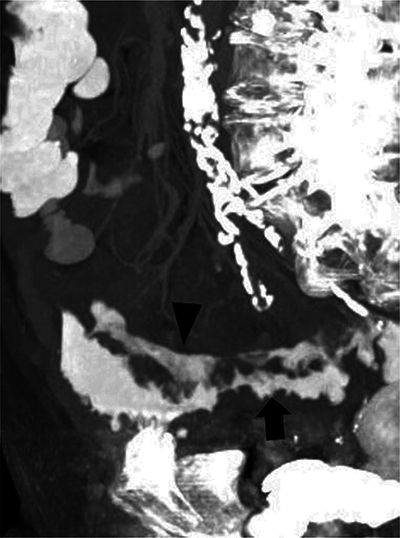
Maximum intensity projection imaging of the abdominal CT: two contrast trajectories are distinguishable, the intramural longitudinal fistula (arrowhead) and the normal intraluminal contrast of the sigmoid (arrow).
